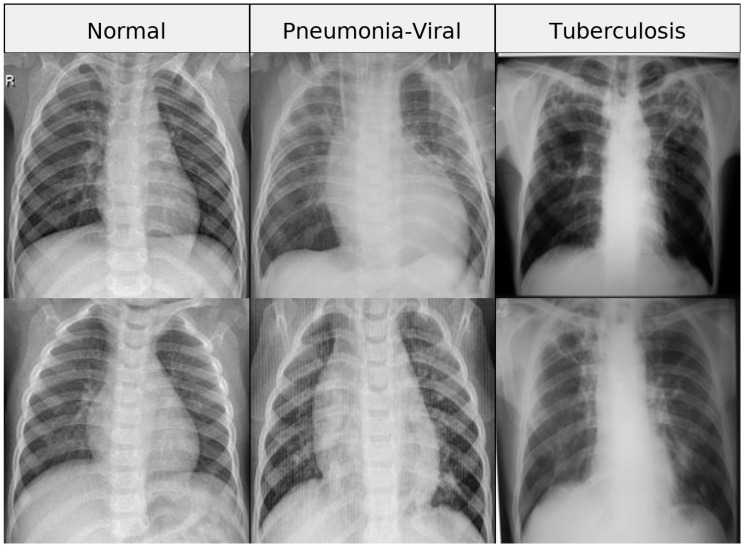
Examples of chest X-rays by class: Normal (left column), Viral pneumonia (center column), and Tuberculosis (right column).
